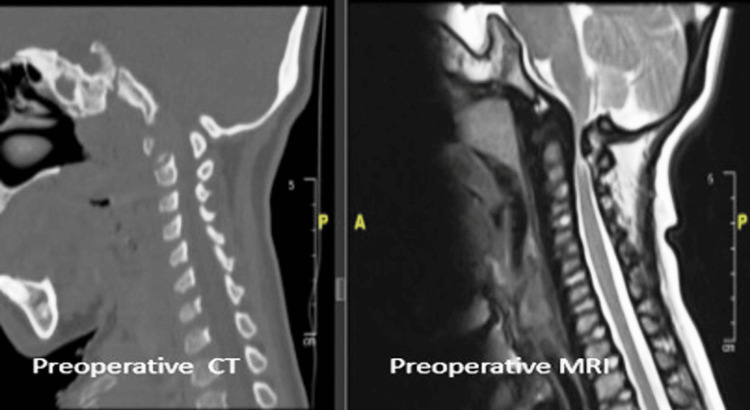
Case 3: Preoperative CT and MRI images of the cervical spine showing cervicomedullary compression and platyspondyly (decreased vertebral body height with increased anteroposterior diameter). AP: Anteroposterior.
